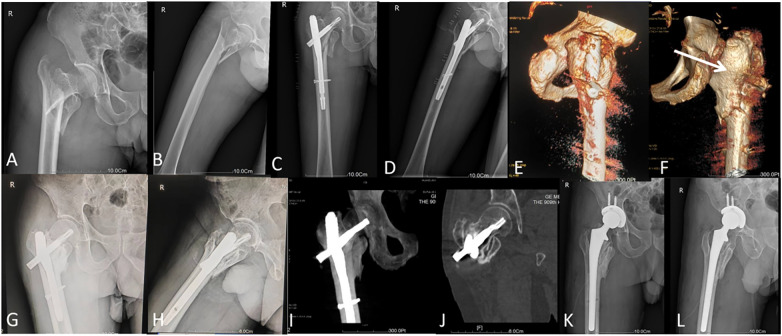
Conventional fixation case: A 68-year-old male presented with a right A2.3 intertrochanteric fracture complicated by type 3 coronal fragments (A,B) following low-energy trauma. Conventional closed reduction with PFNA was performed via fracture table traction (C,D). Postoperative 3D-CT reconstruction revealed inadequate coronal fragment stabilization (E,F), and the arrows indicating posteriorly displaced coronal fracture fragments and suboptimal implant entry through the fracture line. At the 12-month follow-up, radiographic evidence revealed nonunion with catastrophic implant fracture (G–J), resulting in revision surgery with conversion to total hip arthroplasty (K,L).
